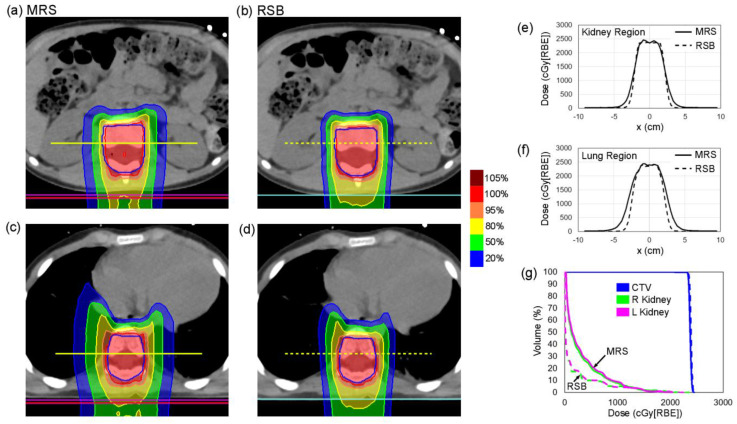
(a–d) CT and isodose distributions for CSI plans produced using the MRS and RSB approaches in a single patient at the level of the (a,b) kidneys and (c,d) lungs. (e,f) Lateral dose profiles extracted at locations indicated by yellow lines in (a–d) at the level of the (e) kidneys and (f) lungs. (g) Dose volume histogram (DVH) curves for the CTV and the left and right kidneys.
